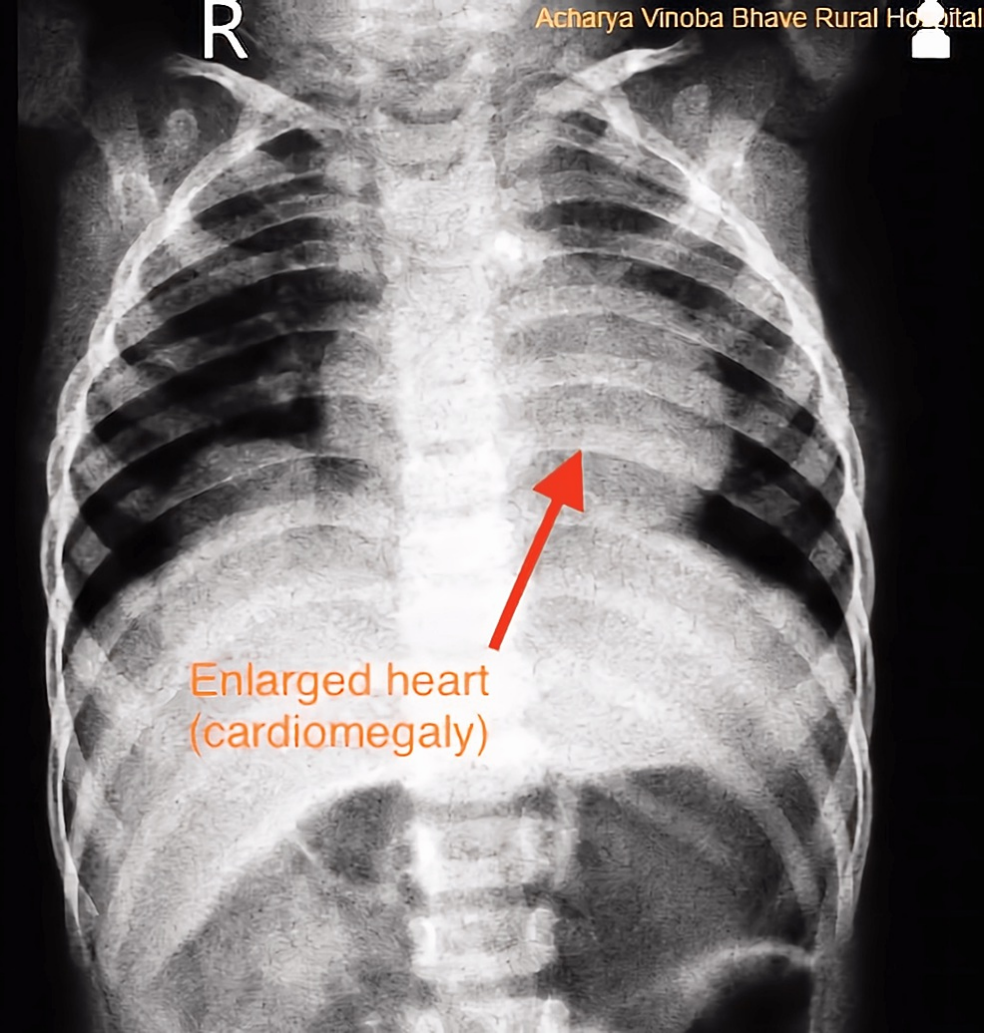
Chest X-Ray Showing Cardiomegaly The image has been taken by the authors.
